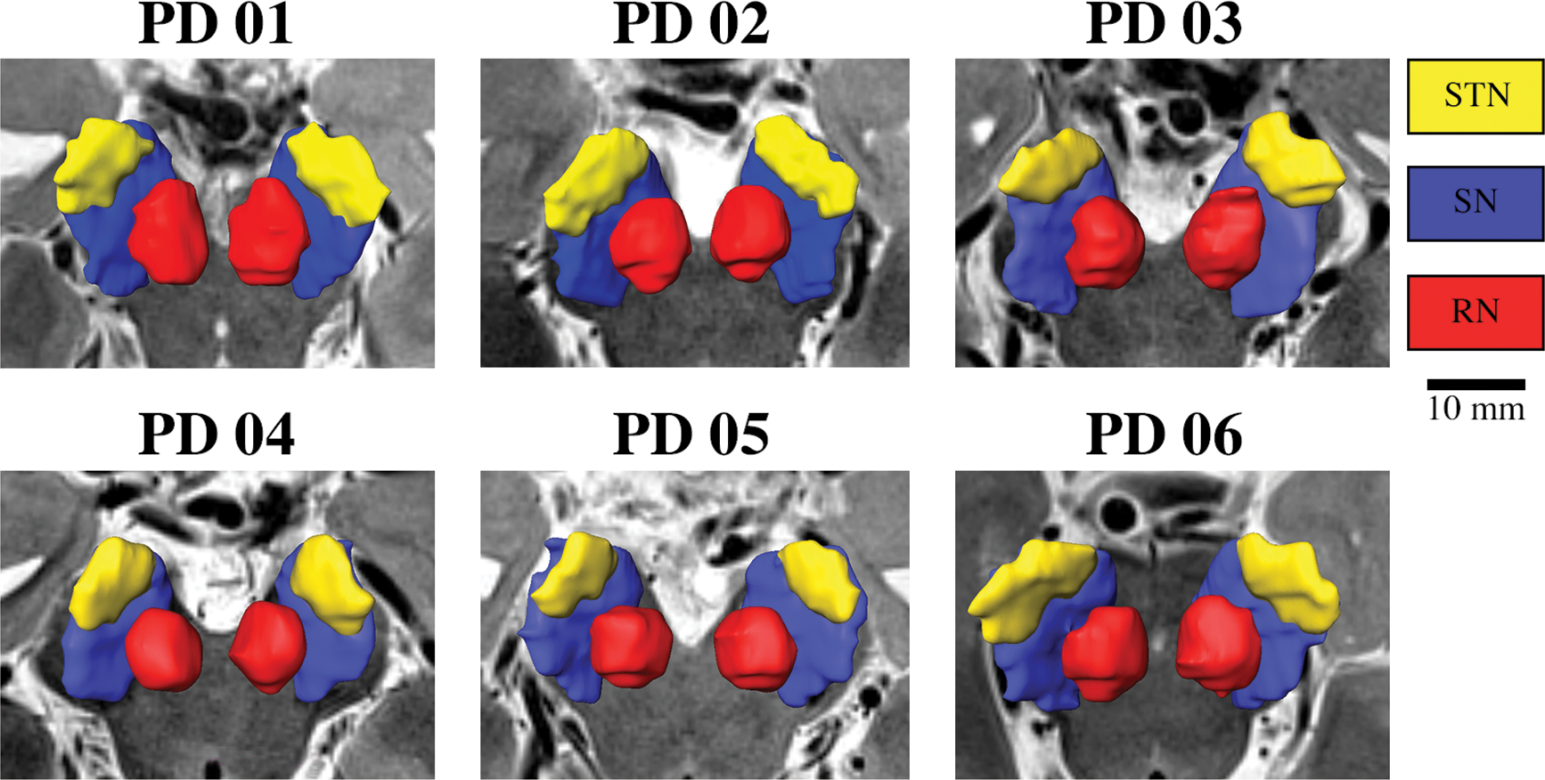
Example of anatomical 3D reconstruction of the STN, SN and Red nuclei. Anatomical 3D reconstruction of the STN, SN and Red nuclei of six different patients imaged with 7T MRI. Note the variability in the shape, size and location of the structures across individuals.
